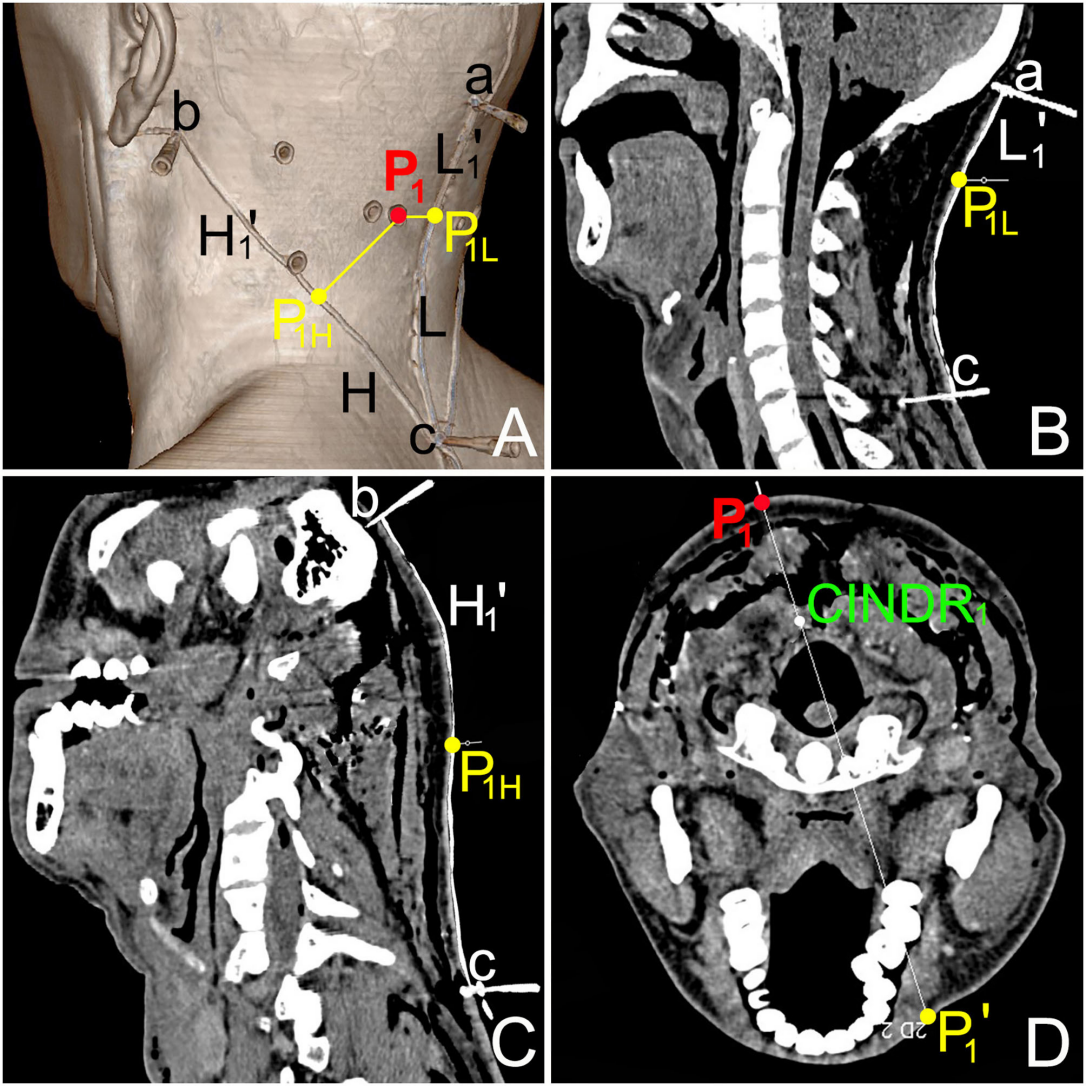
CT images of the center of intramuscular nerve dense region (CINDR_1_) of left rectus capitis posterior minor. (A) Spiral CT three-dimensional reconstruction image shows the body surface projection position and design reference line of the CINDR. Point a is the external occipital protuberance, point b is the mastoid process, and point c is the spinous process of the 7th cervical spine. P_1_ is the body surface projection point of CINDR_1_ of rectus capitis posterior minor. ac line = L reference line, a-P_1L_ = L_1_'; bc = H reference line, and b-P_1H_ = H_1_'. (B) The length of the L reference line and L_1_' line on the sagittal section through the ac line. (C) The length of the H reference line and H_1_' line on the cross-section passing through the bc line. (D) The depth of CINDR_1_, measured on the cross-section through P_1_.
